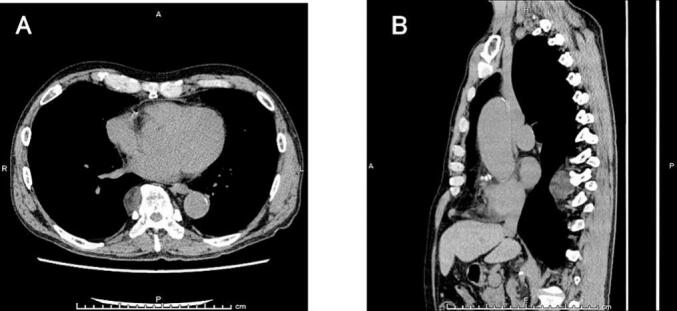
Chest CT image showing a well-encapsulated tumor 3 cm in diameter with a heterogeneous interior adjacent to the right side of the Th8 to Th10 thoracic vertebrae (A: axial mediastinal window, B: sagittal mediastinal window).
